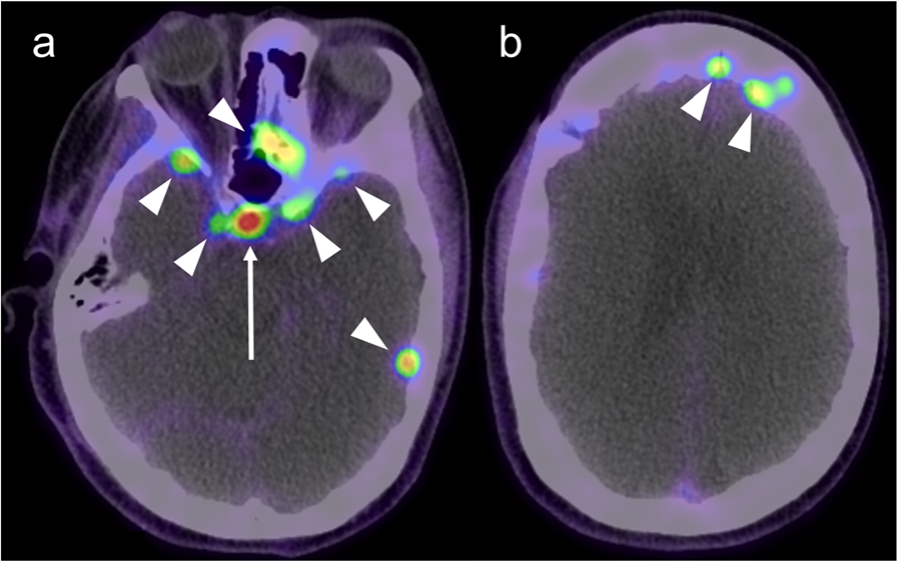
Post-contrast MRI (a) and GaTate-PET (b) in a patient with previous surgery for meningioma. A small enhancing nodule related to the falx cerebri (arrows) demonstrates GaTate-avidity, consistent with meningioma. In contrast, the more diffuse dural thickening (arrowheads) does not demonstrate GaTate uptake, and is thus consistent with post-operative change rather than en plaque meningioma
